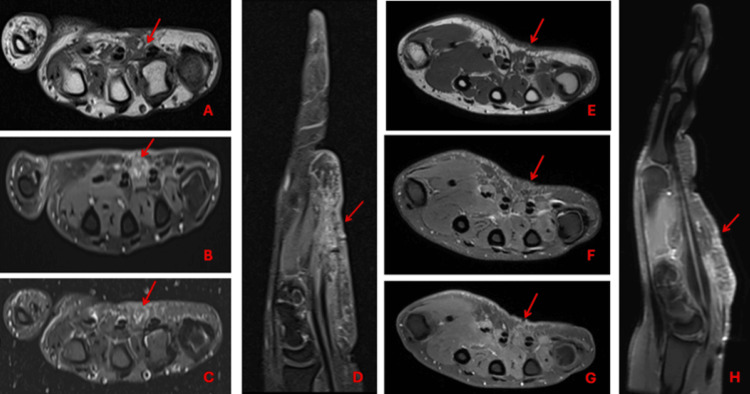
Postoperative MRI of the hand done at one month showing postsurgical changes (red arrows) on axial T1 (A), post-gadolinium-enhanced axial T1 fat-suppressed (B), axial T2 fat-suppressed (C), and sagittal PD fat sat (D) images. Postoperative MRI of the hand done at three months showing postsurgical changes and scarring (red arrows) on the axial T1 fat-suppressed (E), axial PD fat-suppressed (F), post-gadolinium-enhanced axial (G), and sagittal (H) images MRI: magnetic resonance imaging; PD: proton density
